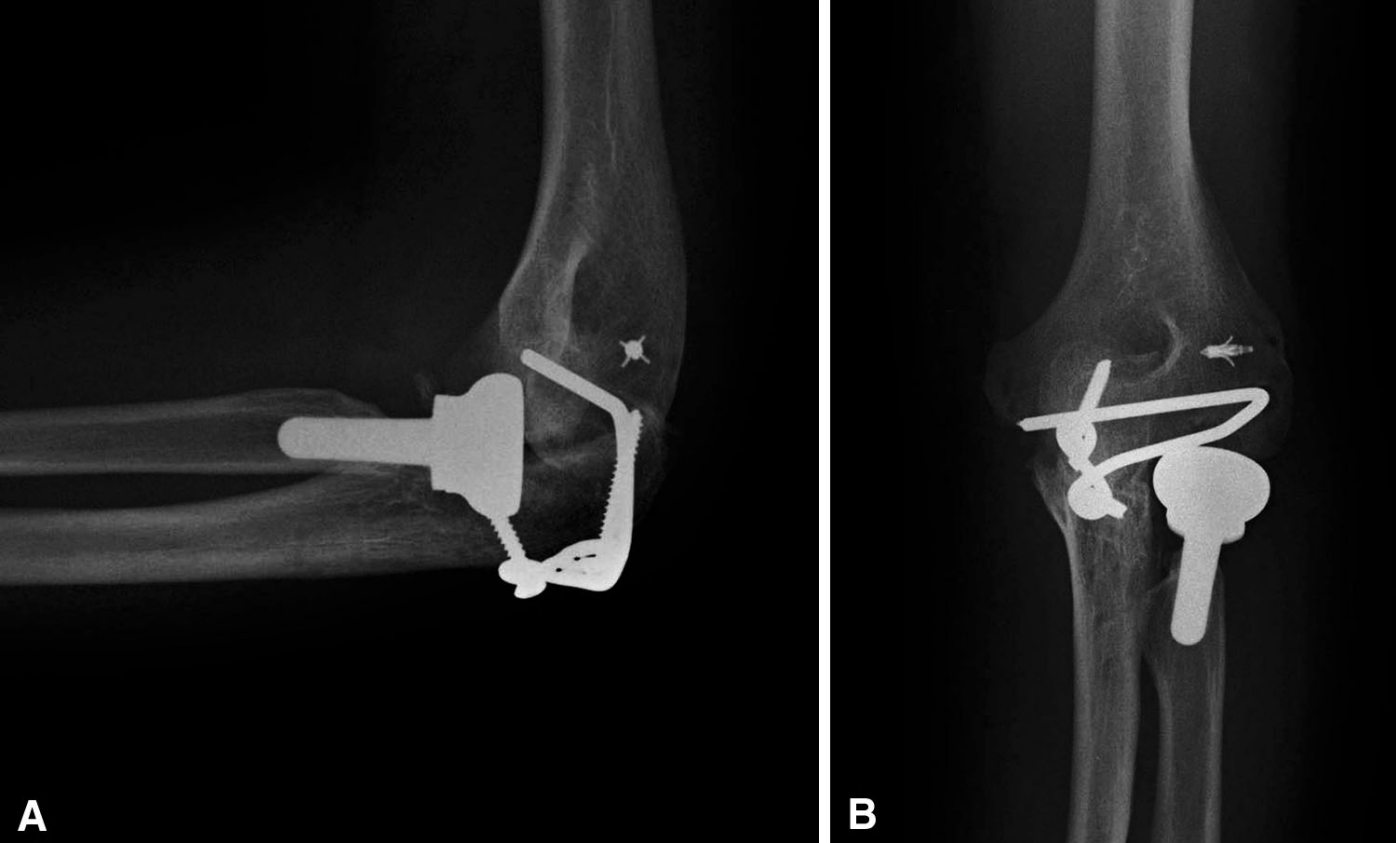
(A) Lateral and (B) AP radiographic views of the joint stabilizer are shown.
